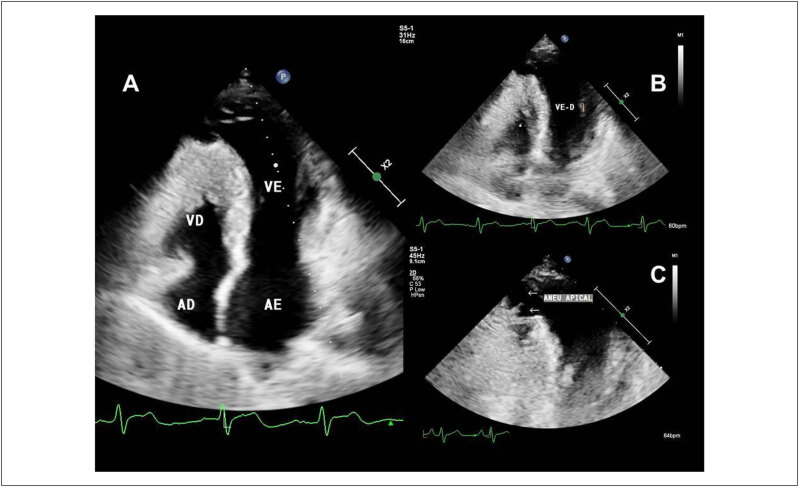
Imagens ao ecocardiograma transtorácico de paciente com MCH evidenciando aneurisma apical ao corte apical 4 câmaras (A e B) e apical 2-câmaras (C). AD: átrio direito; AE: átrio esquerdo; VD: ventrículo direito; VE: ventrículo esquerdo. Fonte: Arquivo pessoal dos autores.
